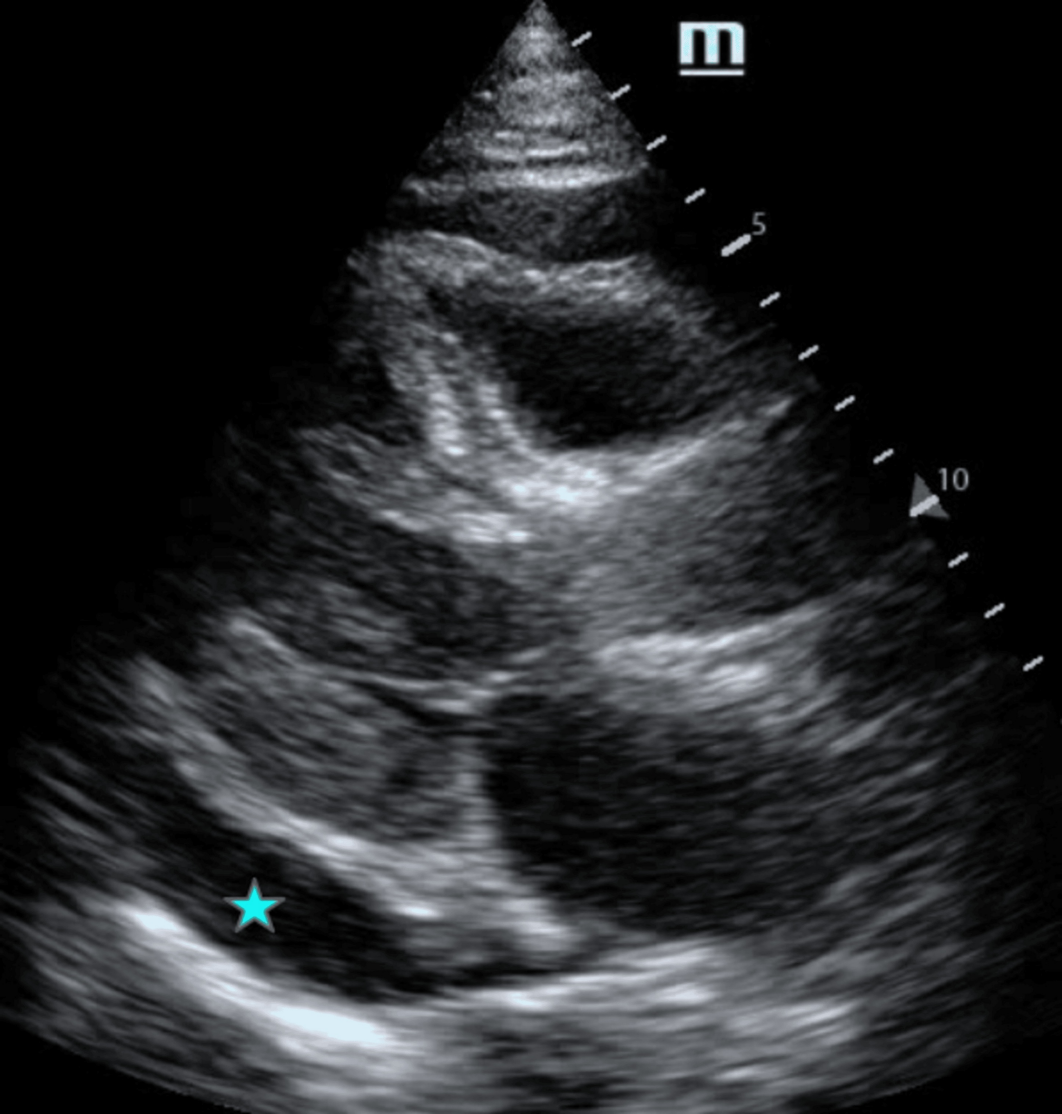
Parasternal long-axis view demonstrating a circumferential pericardial effusion Transthoracic echocardiography in the parasternal long-axis view demonstrating a circumferential pericardial effusion (blue asterisk) with evidence of right-sided chamber compression.
